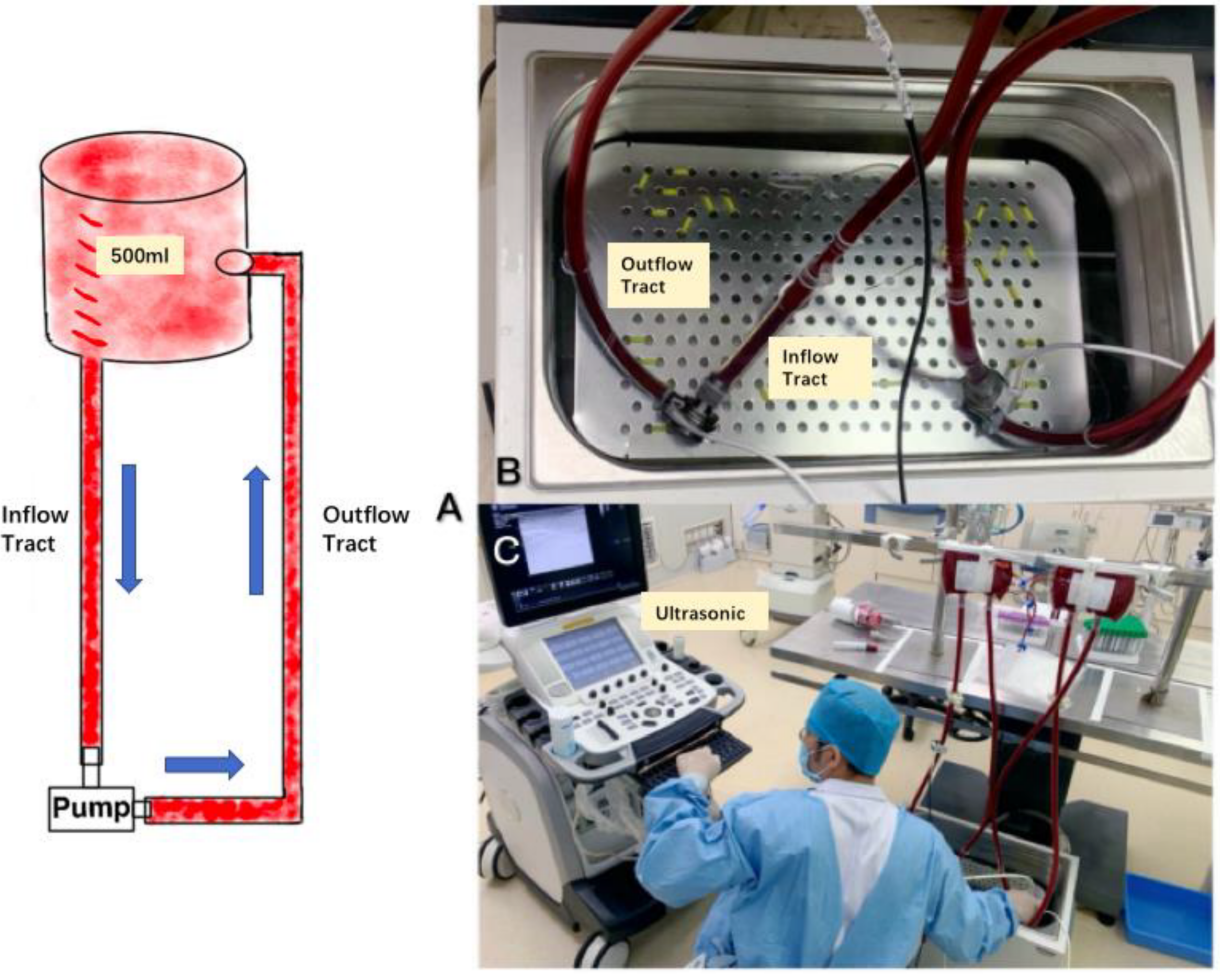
(A) Shows the *in vitro* experimental line, (B) shows the pump placed in a 37°C water bath to maintain a constant temperature, and (C) shows the number of bubbles generated within the diagnostic line through ultrasound.
